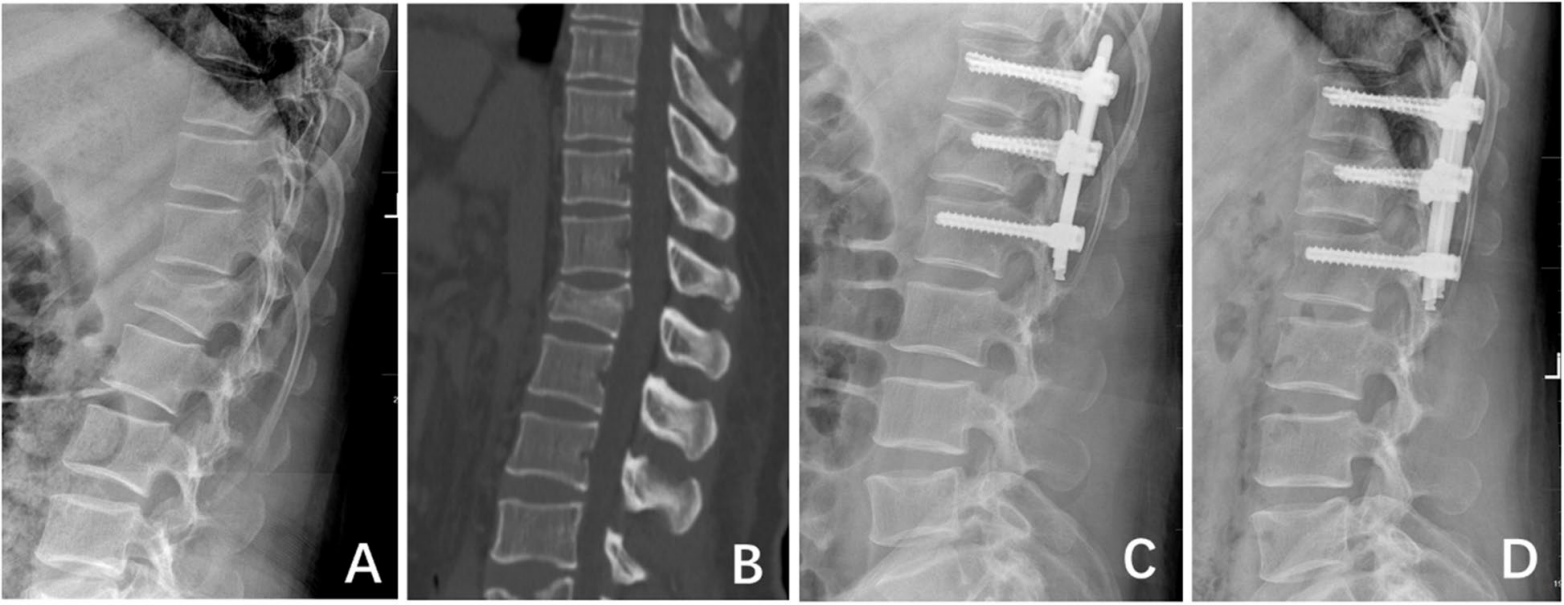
Lateral (A) radiograph and CT (B) of a 35-year-old male with L1 vertebral fracture (AO type B2). Receiving percutaneous short segment pedicle screw fixation without screwing of the fractured vertebra with O-arm navigation and the Cobb angle and AVH% get restoration one week (C) and one year (D) after the surgery
